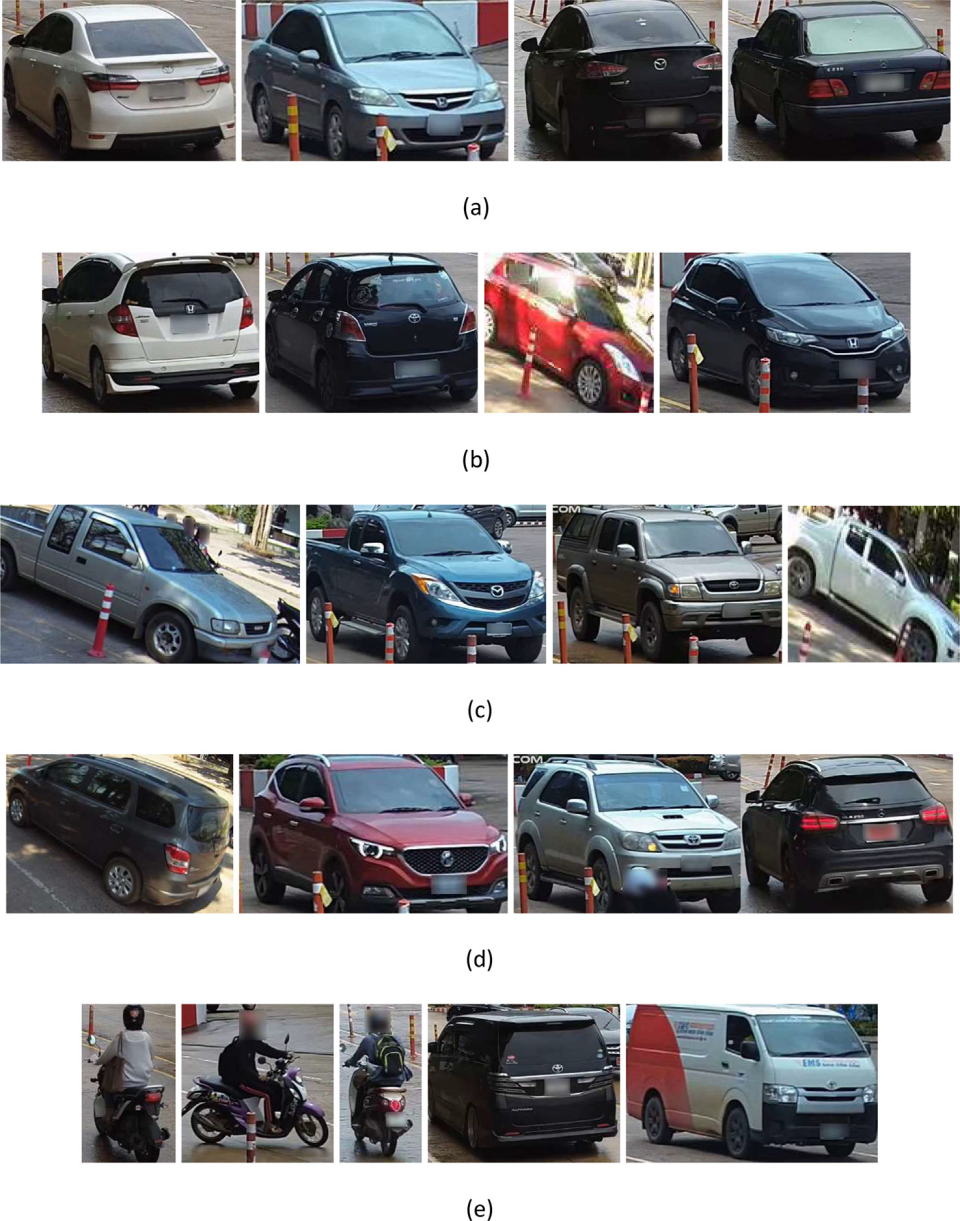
Examples of the VTID dataset: (a) Sedan, (b) Hatchback, (c) Pick-up, (d) SUV, and (e) Other vehicles.
